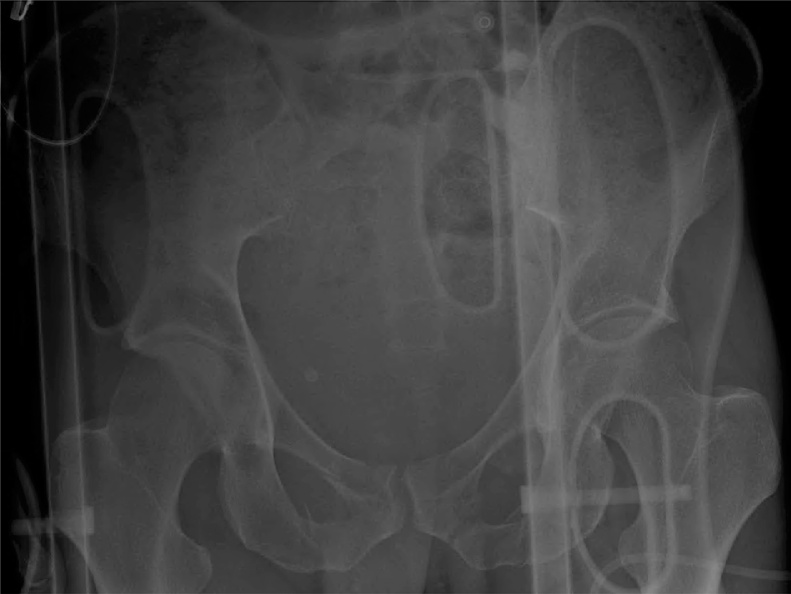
Pelvic anteroposterior X-rays taken before surgery.
